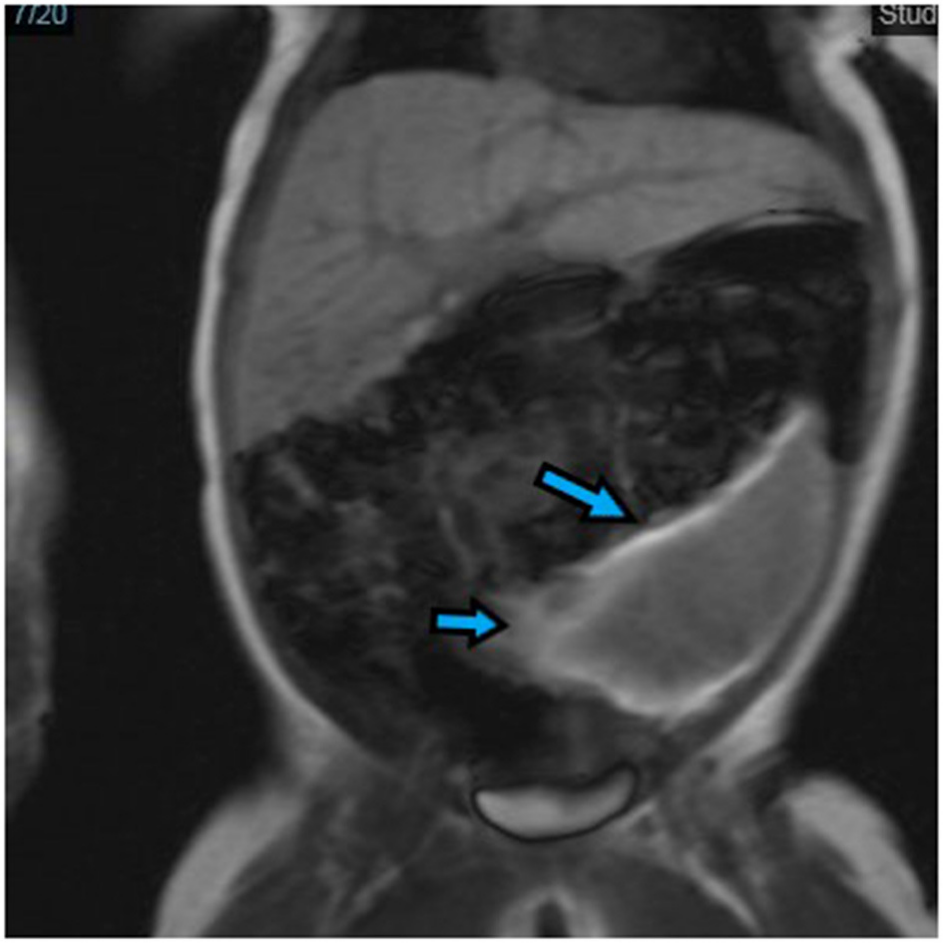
Frontal view of magnetic resonance imaging (MRI) of the abdomen. Wandering spleen (arrow) presented as a well-defined semilunar-shaped structure in the left lumbar region with the descending colon lateral and the left kidney posterior in the lower abdomen. It is seen anterolateral to the small bowel and just beneath the anterior abdominal wall muscle.
